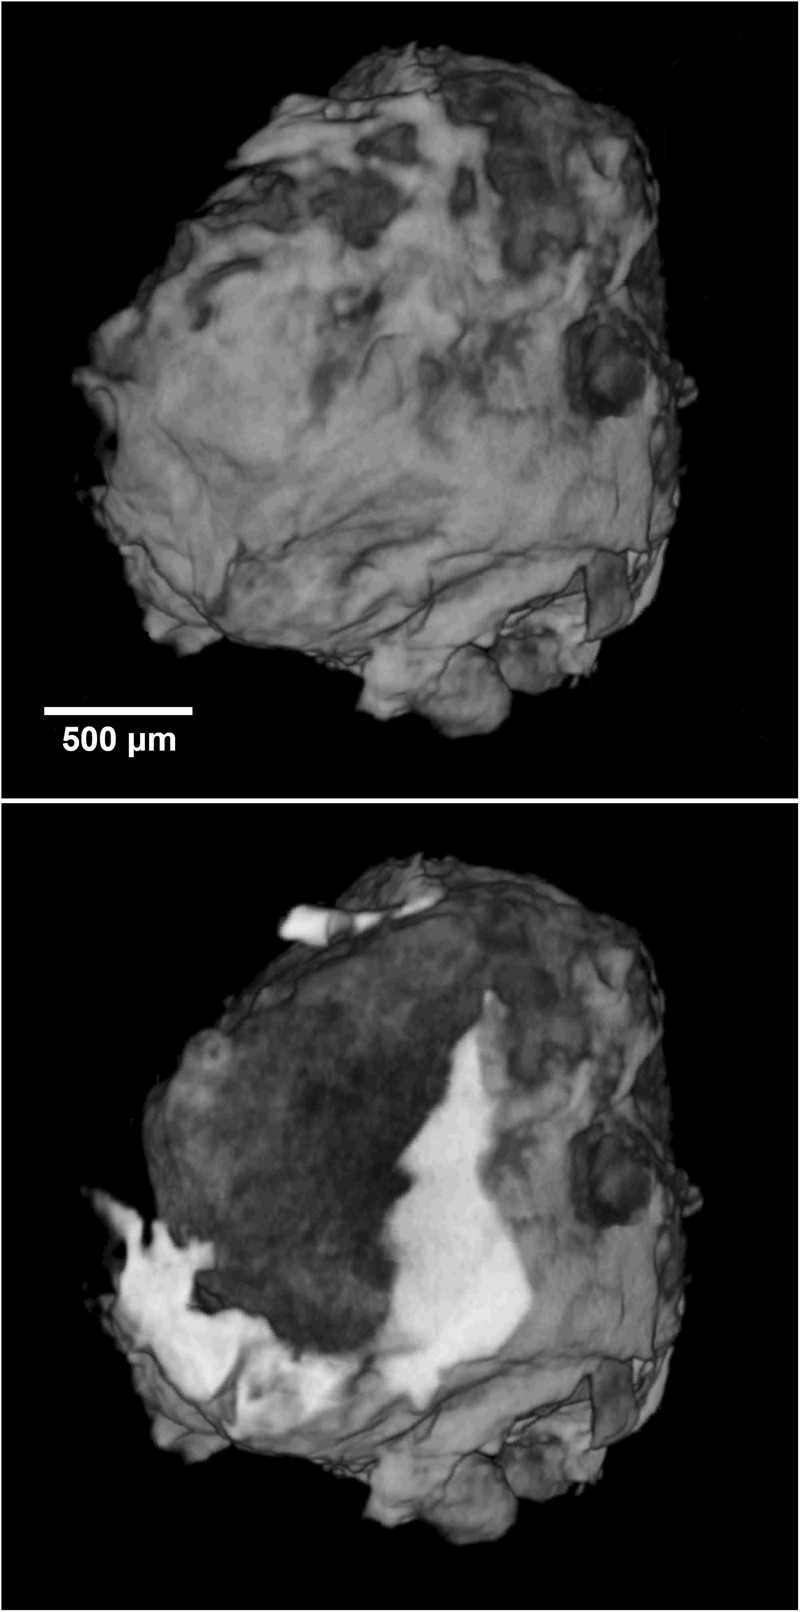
Micro-computed tomographic images (at different orientations) of the entire grain (labeled number 126). Light grey and dark grey regions refer to metallic (AlCu, AlCuFe, AlFe, and AlNiFe minerals) and silicatic portions.
